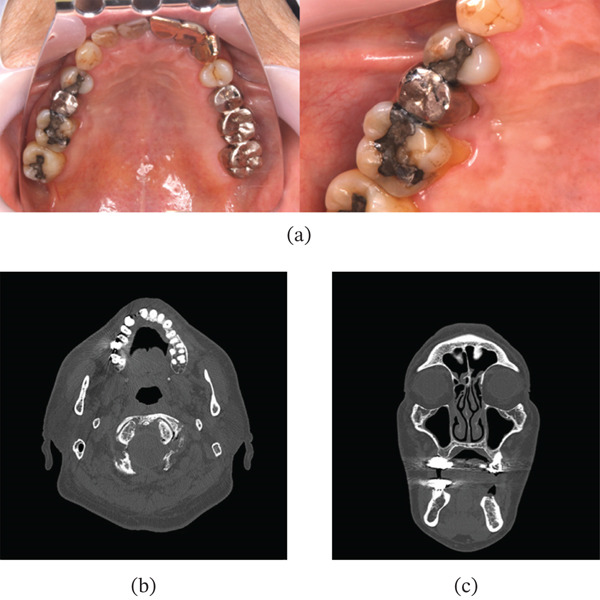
Postoperative intraoral photograph and CT images. (a) Intraoral photographs obtained 2 years postoperatively show no evidence of tumor recurrence. (b) Axial and (c) coronal CT images obtained 1 year postoperatively demonstrate only bone resorption attributable to peripheral ostectomy.
